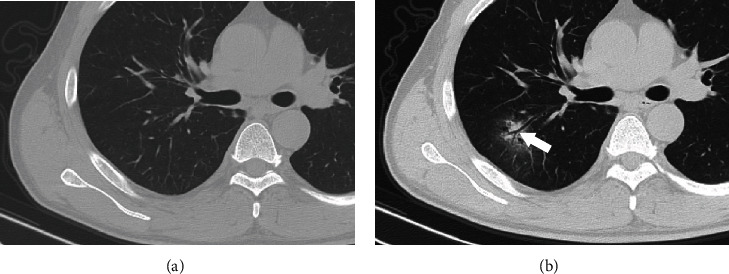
A 51-year-old man with unknown exposure history presented with fever and dry cough for 2 days. (a) The first chest CT scan shows normal findings. (b) Four days later, the second chest CT scan shows patchy ground-glass opacity with consolidation in the posterior segment of the right upper lobe. Bronchiectasis (arrow) could be seen in the lesion.
